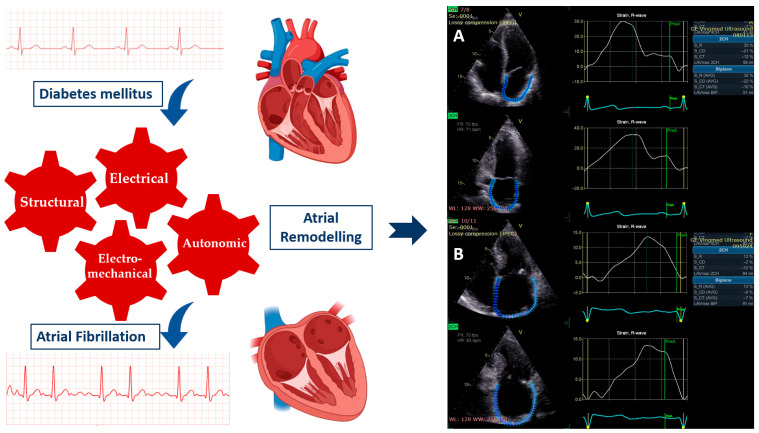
Left side. Patients with diabetes mellitus exhibit atrial remodeling at the structural, electrical, electromechanical, and autonomic levels. Atrial myopathy is associated with an increased risk of atrial fibrillation. Right side. (A) The three functions of the left atrium were evaluated by speckle tracking echocardiography in a patient without diabetes. (B) The patient with diabetes has lower values for all three left atrial functions and higher values for left atrial volume.
